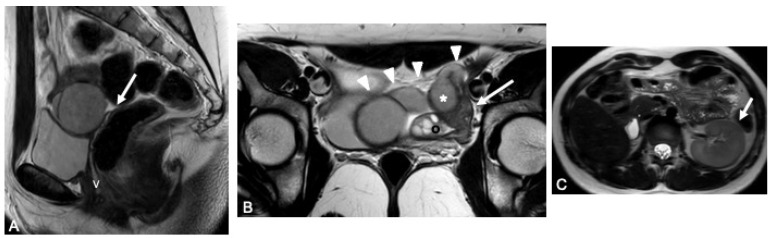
14-year-old teenage girl with primary amenorrhea, pelvic pain. (A) Sagittal Τ2W MR image of the pelvis demonstrates absence of a normal uterus and cervix (arrow). In the expected location of the vaginal canal (v) a thin fatty band is observed. (Β) Axial oblique Τ2W MR image of the pelvis in the same patient shows a left uterine remnant (arrow). A distended with hemorrhagic fluid endometrial cavity (asterisk) and associated hematosalpinx (arrowheads) are observed, indicative of the presence of functional endometrium. Closely associated normal volume ipsilateral ovary (o). (C) Axial Τ2W MR image of the upper abdomen in the same patient shows absence of the right kidney. Normal renal location and hypertrophy is shown on the left (arrow). Findings are consistent with Müllerian agenesis with unilateral uterine remnant with functional endometrium.
